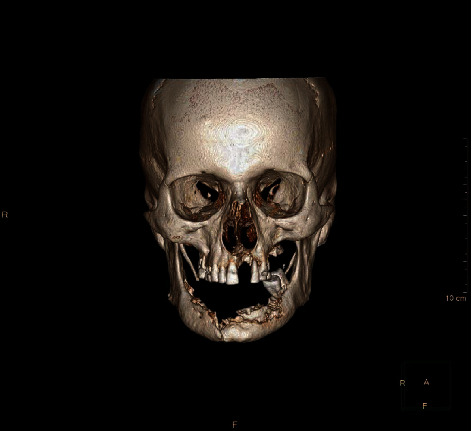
Preop CT scan showing right parasymphysis and dentoalveolar factures.
